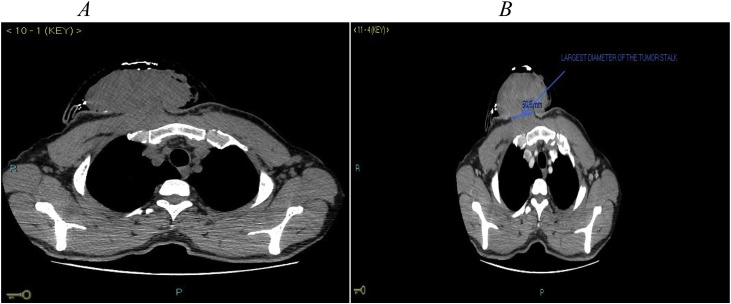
A: Axial view of non-contrast CT-scan showing a tumour with calcified capsule and with no evidence of bony erosion. B: Axial view of a contrast CT-scan showing multi-dense tumour with a broad stalk and loss of subcutaneous plane at the insertion of the stalk.
